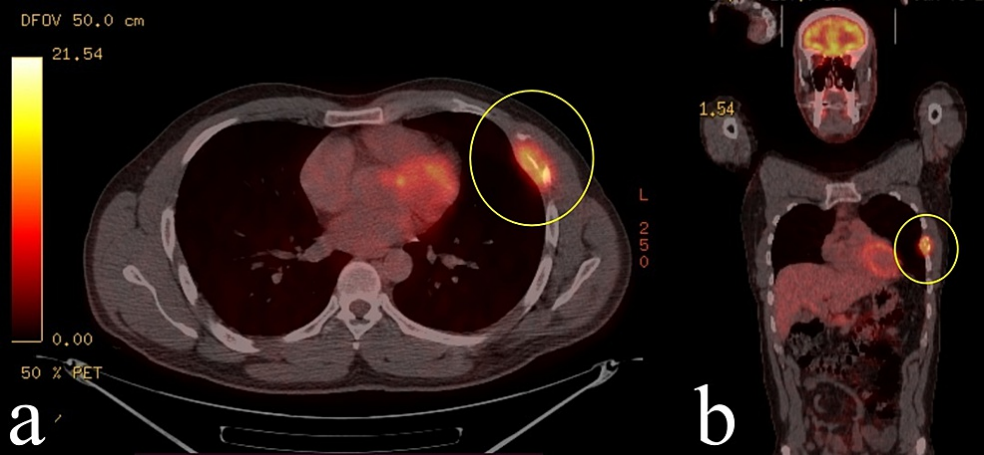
Eosinophilic granuloma involving the fifth and sixth ribs with pathologic 18F-FDG uptake (SUVmax: 6,8) on PET/CT axial (a) and coronal (b) images (yellow circle) The first admission was in 2014. FDG, Fluorodeoxyglucose; SUVmax, maximum standardized uptake value; PET/CT, positron emission tomography/computed tomography.
